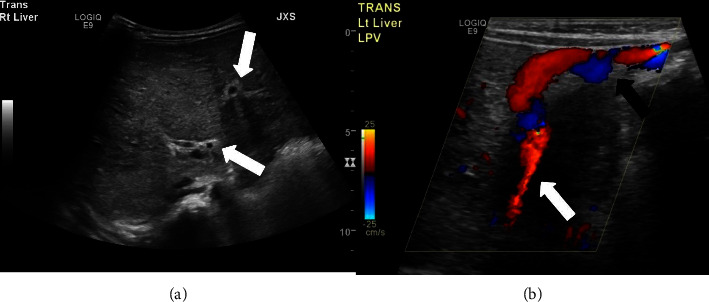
(a) Transverse grayscale image of the right hepatic lobe shows diffuse, heterogeneous, and mildly coarsened echotexture with periportal hyperechogenicity and thickening (white arrows) suggesting fibrosis. (b) Transverse ultrasound image with color Doppler shows the left portal vein (white arrow) with recanalized umbilical vein extending anteriorly to just beneath the skin (black arrow).
